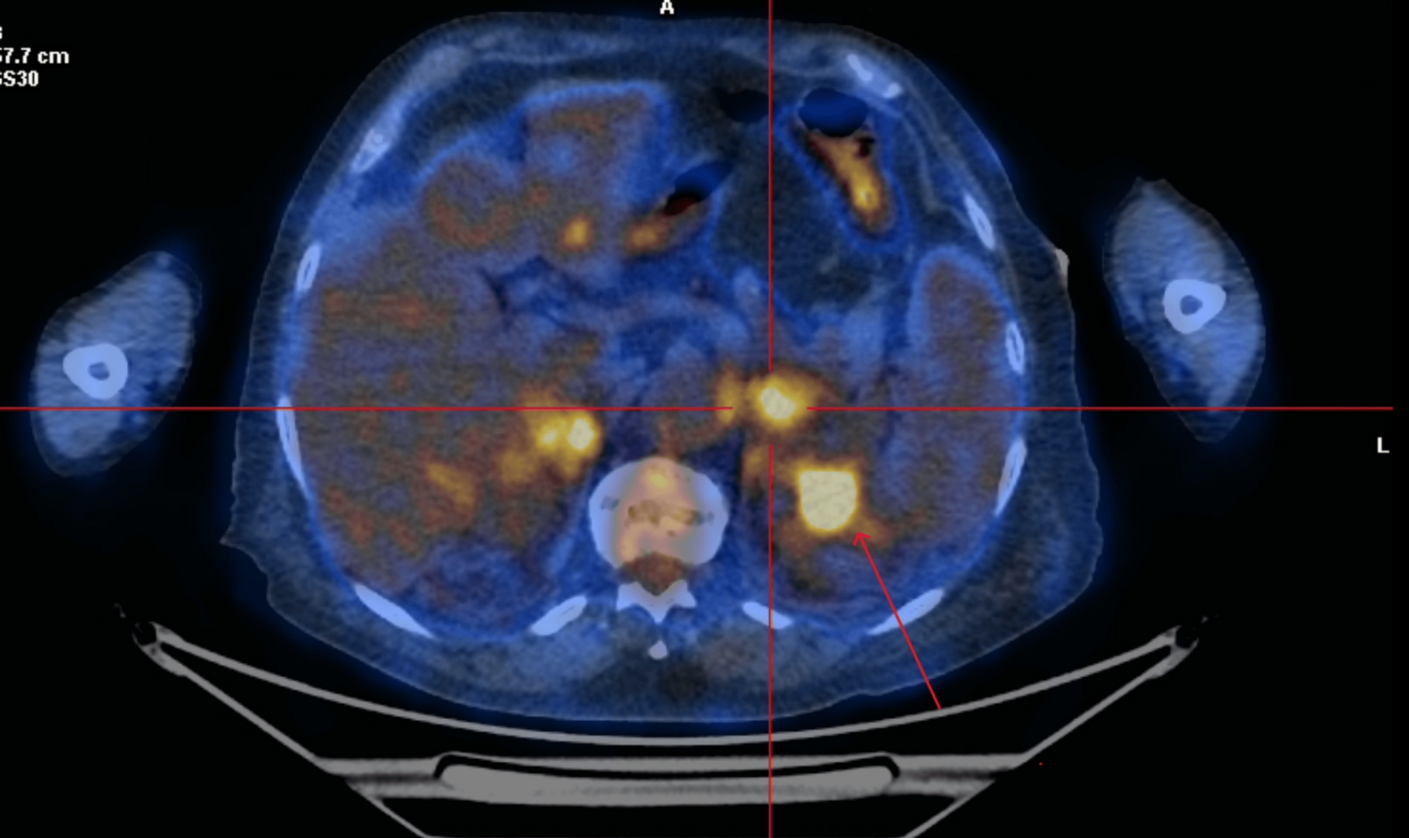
PET CT scan image of the kidney (axial view) Positron emission tomography (PET) CT scan image of the kidneys showing thickening of soft tissues in the region of the renal fascia.
